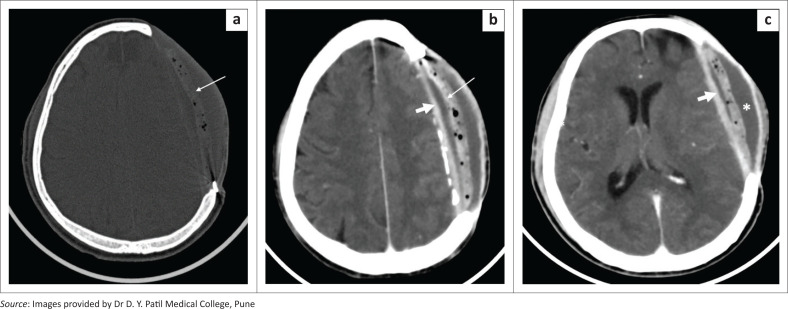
Cranioplasty flap infection. Axial bone window (a) and contrast-enhanced soft tissue window CT images (b, c) in a patient with a left frontoparietal craniectomy reveals an acrylic cranioplasty implant with small air foci (a, b, long arrows). Adjacent epidural (b, c, thick arrow) and subgaleal (c, asterisk) fluid collections along with thickening and enhancement of the meningogaleal complex is seen.
